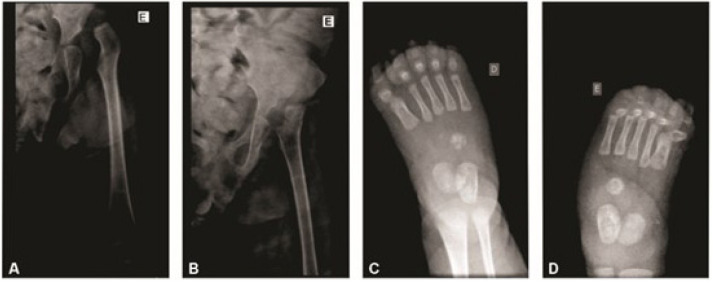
Radiographs of patient 1. (A-B) Left hip showing femoral head dislocation; and right (C) and left (D) feet showing structural changes in the hindfoot, midfoot, and forefoot regions.
